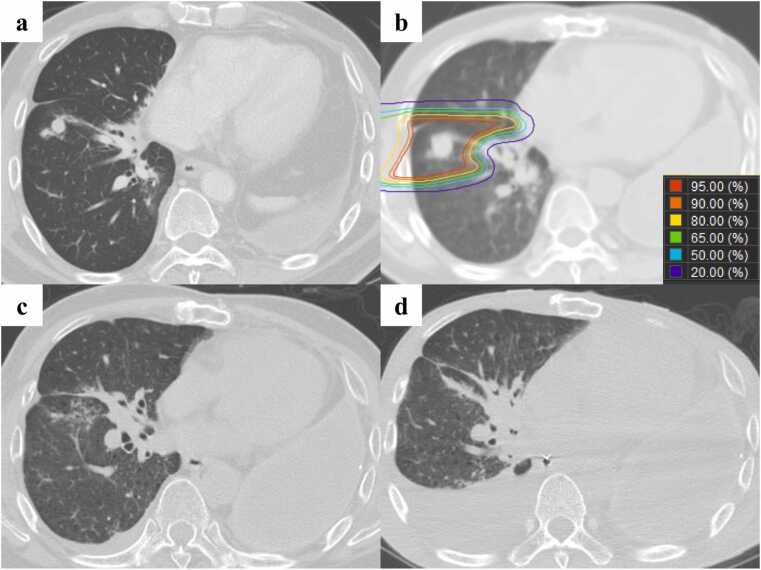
Computed tomography images of case 1 at the time of and after the second proton beam therapy round. a) A newly developed squamous cell carcinoma in the right S9 at 53 months after the initial PBT. b) The dose distribution of the second round of PBT delivered to the right S9 tumor. c) Radiation fibrosis without local progression in the irradiated field at 43 months after the second PBT round. d) Bacterial pneumonia in the right lower lobe, which was the cause of death, at 60 months after PBT.
